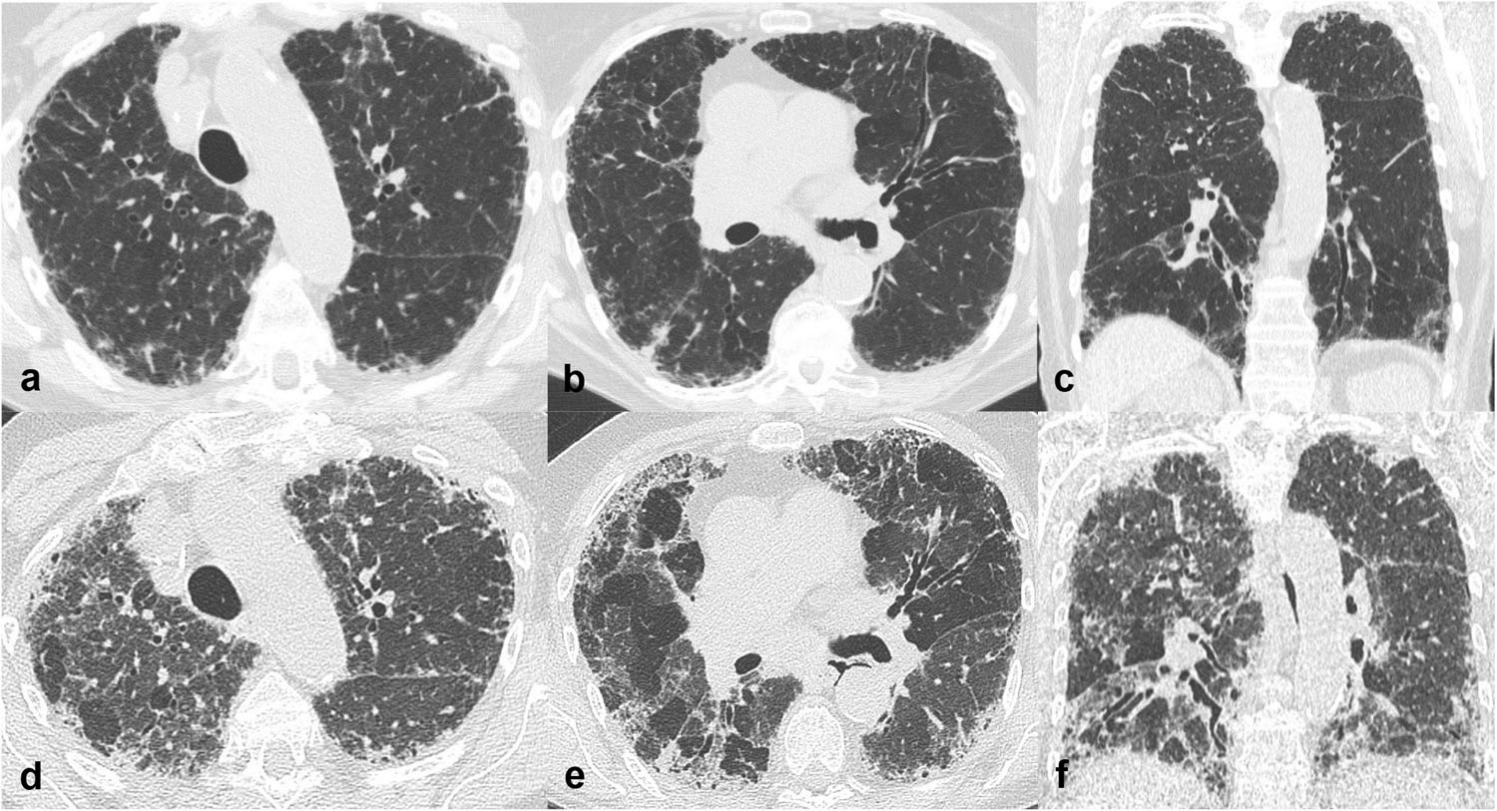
Progressive pulmonary fibrosis (PPF). Axial and coronal computed tomography (CT) images (a–c) showing mild signs of fibrosis without honeycombing in the subpleural areas bilaterally, with mild predominance in the lung bases, suggestive of a probable usual interstitial pneumonia (UIP) pattern in a patient with chronic exposure to pigeons. The multidisciplinary discussion provided a provisional diagnosis of fibrotic hypersensitivity pneumonitis (fHP). Due to worsening dyspnoea, the patient underwent a follow-up CT after 13 months. Axial and coronal CT images (d–f) demonstrate unequivocal disease progression with respect to the previous exam, with increased extent and coarseness of the irregular interstitial thickening and traction bronchiectasis, predominant at the periphery in the upper lobes and more extensive in the mid-lower lungs, associated with marked ‘three-density pattern’ (in inspiratory scans) and lung volume loss. A diagnosis of fHP with progressive behaviour was confirmed, and antifibrotic treatment was initiated
